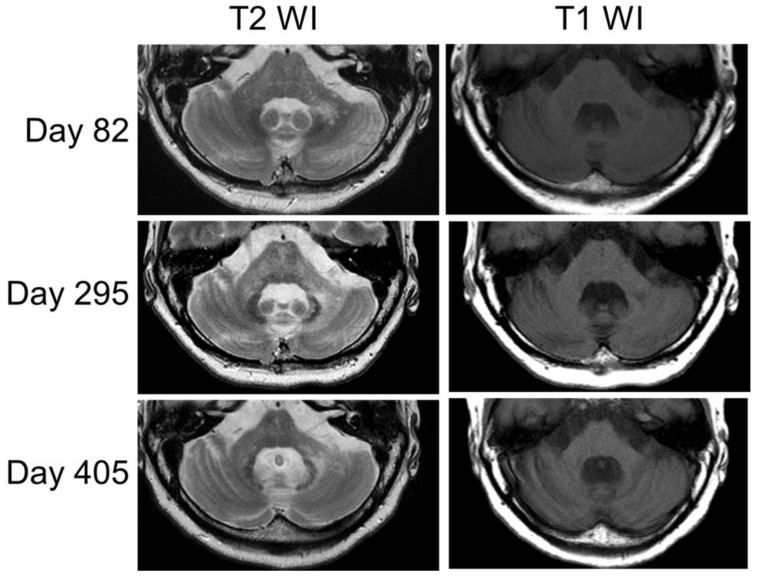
The MRI findings changed over time (days 82, 295, and 405). The left peduncle lesion appeared enlarged on the T2-weighted images, and with this enlargement, atrophy became prominent in both the cerebellum and brainstem on the T1-weighted images.
